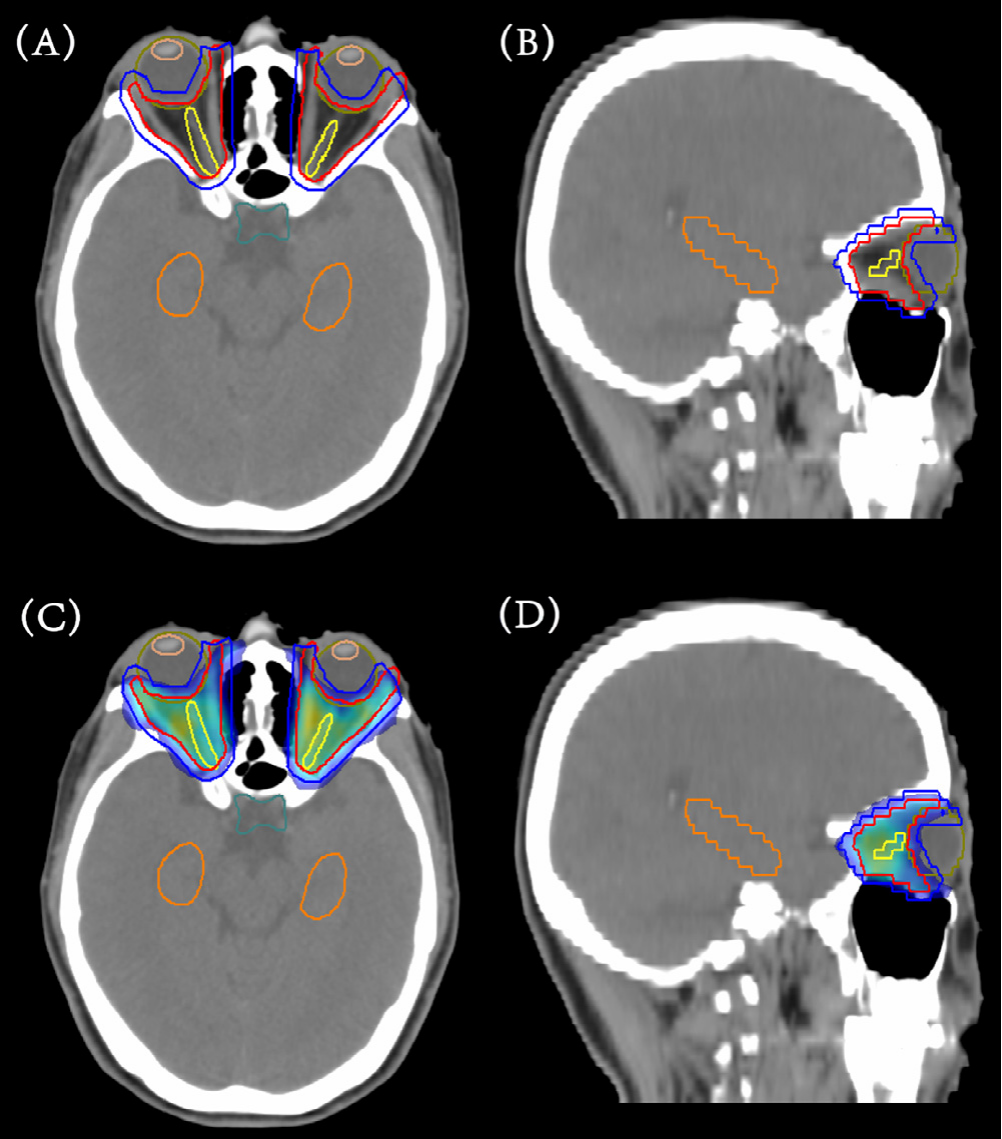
Representative images of a volumetric-modulated arc therapy plan for Graves’ ophthalmopathy. (A) Axial computed tomography (CT) image with clinical target volume delineated in red, planning target volume delineated in blue, and organs at risk. (B) Sagittal CT image with clinical target volume, planning target volume, and OARs. (C) Axial CT image with prescription dose distribution. (D) Sagittal CT image with prescription dose distribution.
